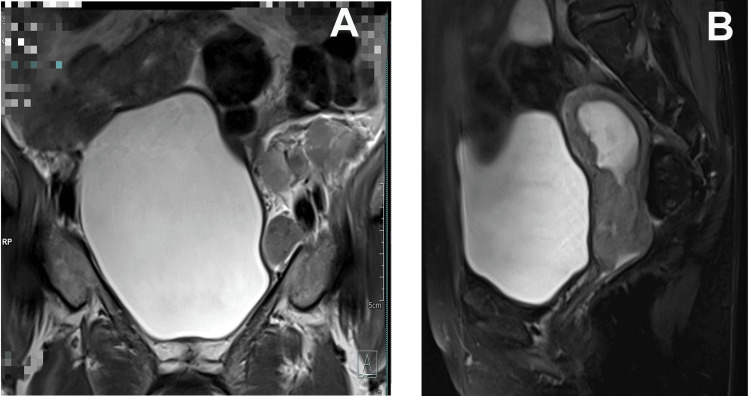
MRI of the pelvis showing (A) a coronal section and (B) a sagittal section. MRI pelvis (A): Coronal section showing metastatic lymphadenopathy in the left external iliac and left obturator stations, forming a nodal mass that abuts the left common and external iliac vessels. Involvement of the right external iliac and obturator stations is also noted. MRI pelvis (B): Sagittal T2 fat-saturated image revealing an irregular circumferential mass lesion involving both the anterior and posterior lips of the cervix, with narrowing of the endocervical canal.
